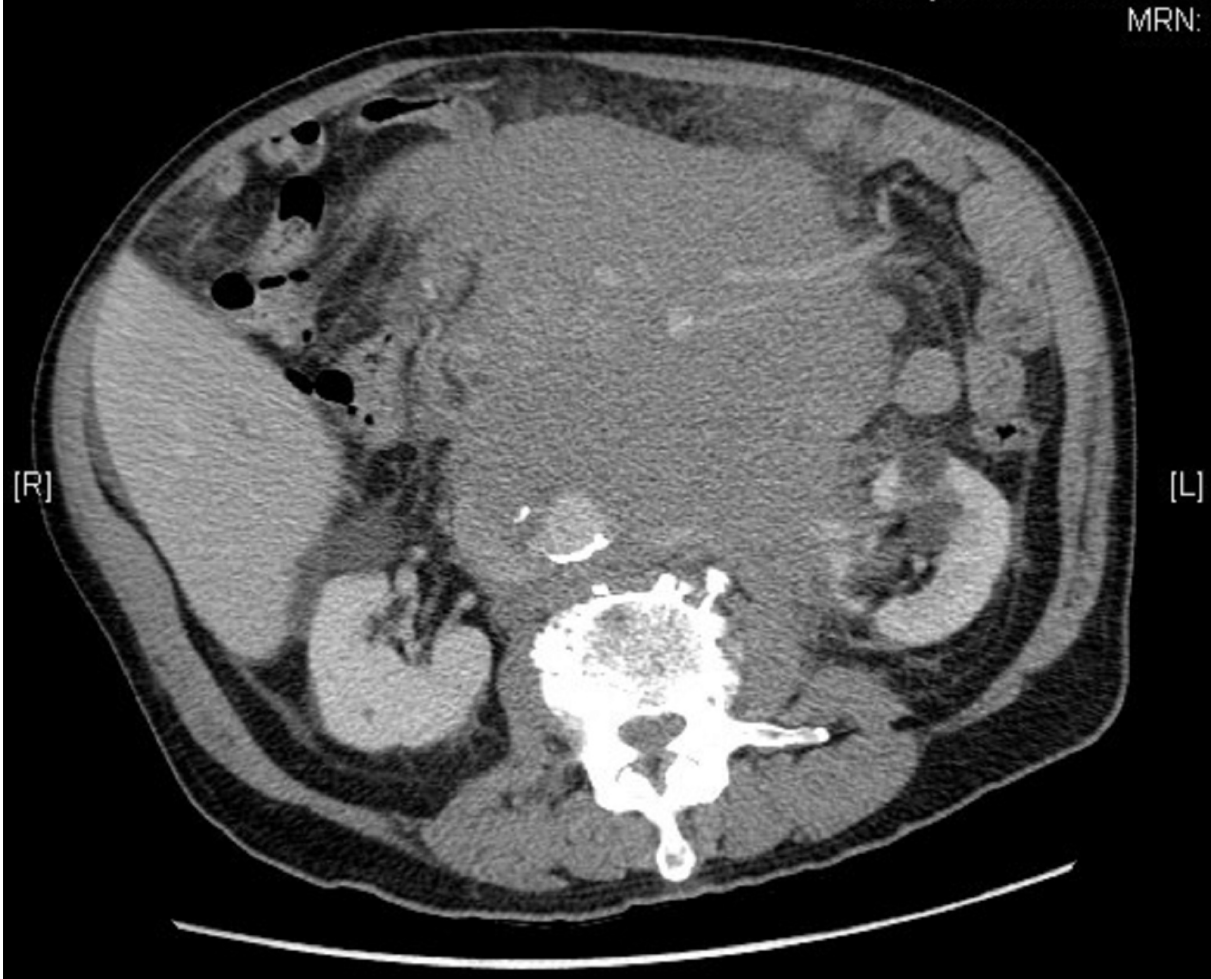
Axial CT showing a large retroperitoneal mass with encasement of the aorta
